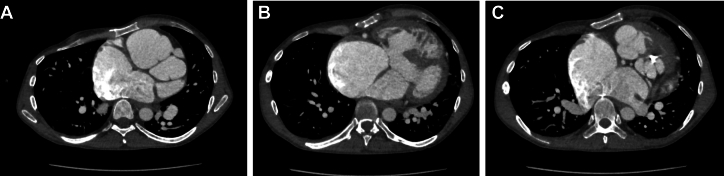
Computed Tomographic Angiography Depicting Variant Coronary Anatomy Computed tomographic angiography delineated the variant coronary artery anatomy. The left circumflex artery originates from the right coronary artery with focal aneurysm of the proximal left anterior descending artery at the first diagonal. (A) The left coronary aneurysm. (B) The large inlet ventricular septal defect. (C) The atrial septal defect and right coronary and left circumflex arteries.
